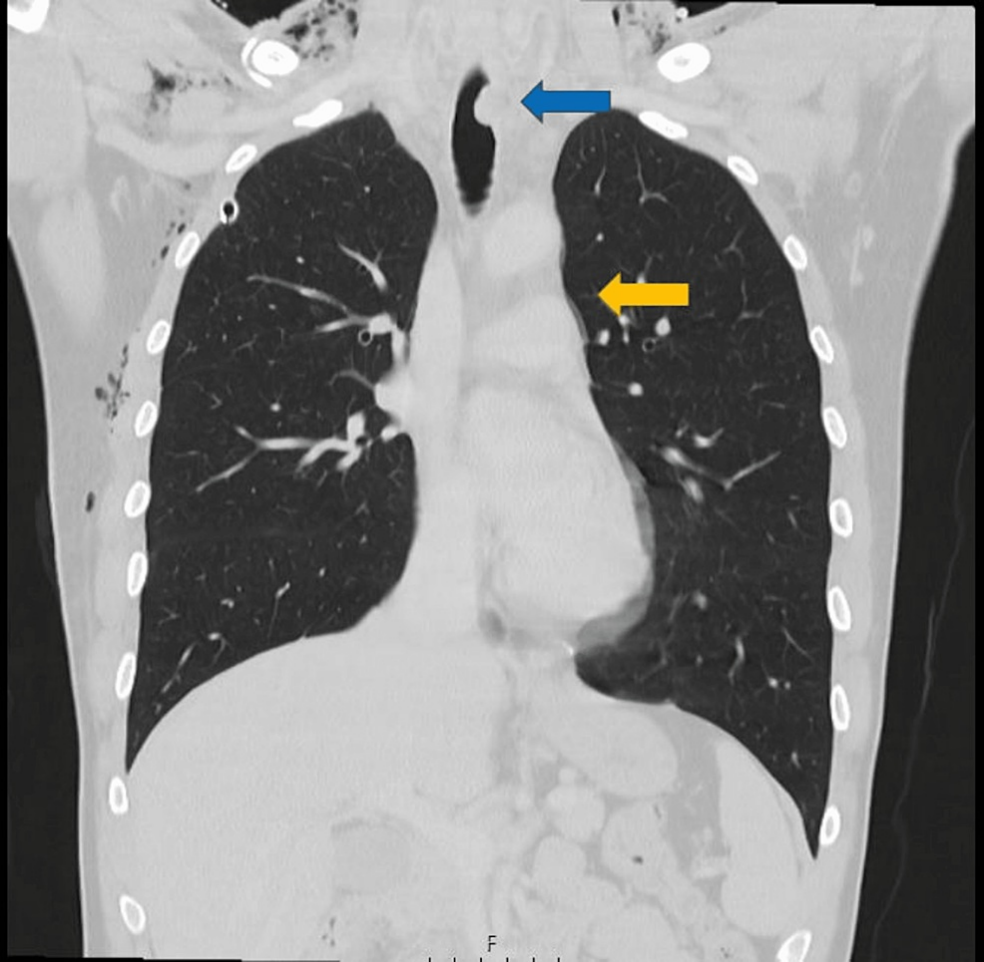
Discharge thoracic CT: Remaining bulging of the left lateral tracheal wall (blue arrow), subcutaneous emphysema and pneumomediastinum reduction (yellow arrow)
